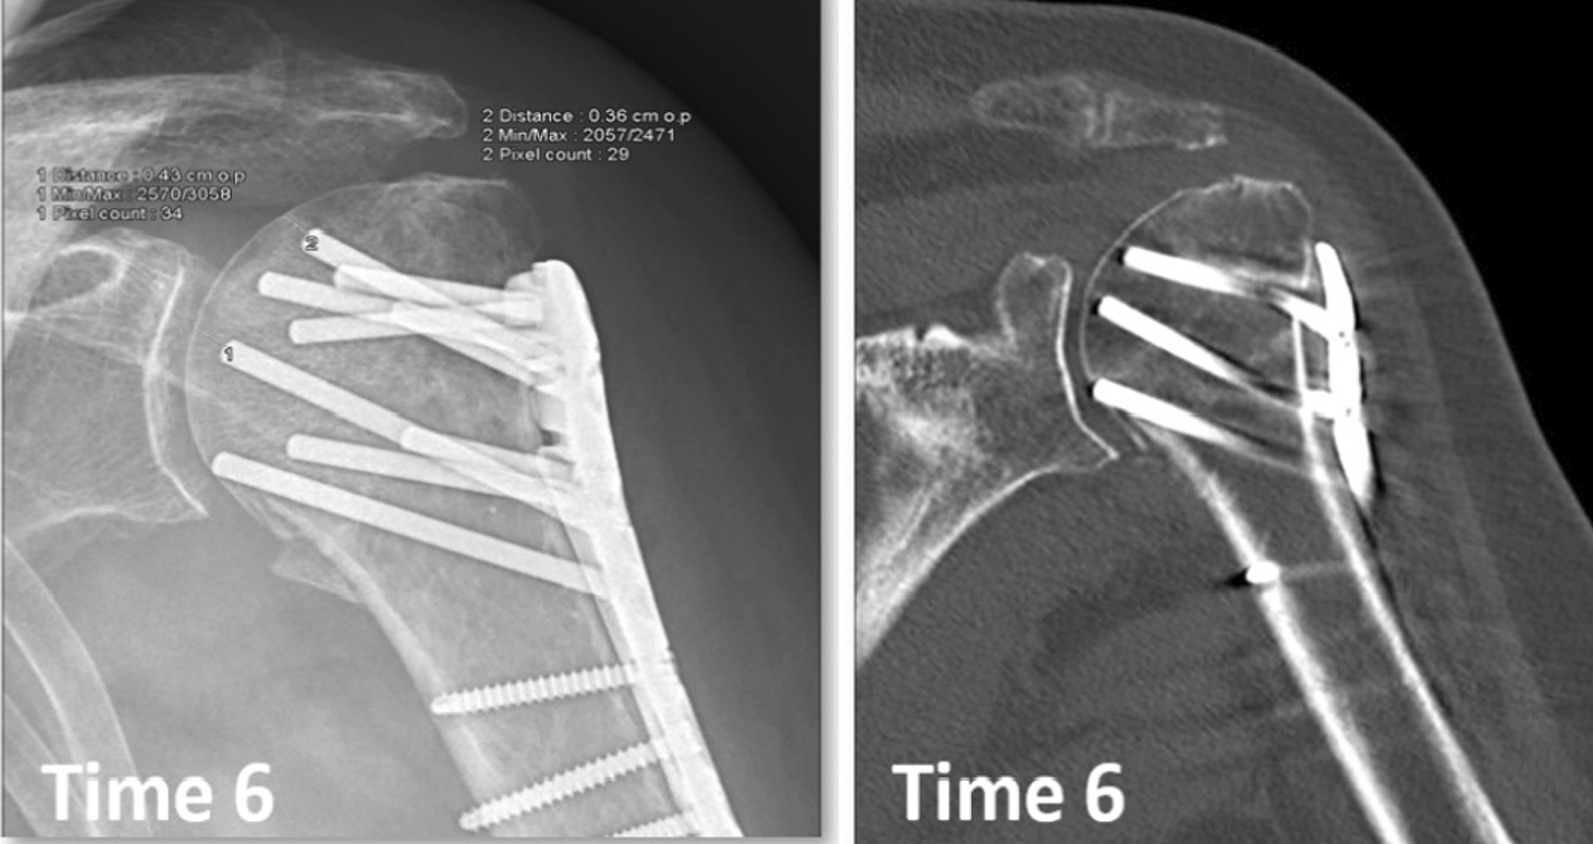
Proximal humeral fracture operated with an ALPS-PHP. At 6 months, radiograph gives suspicion of peg perforation, and CT scan confirms this
